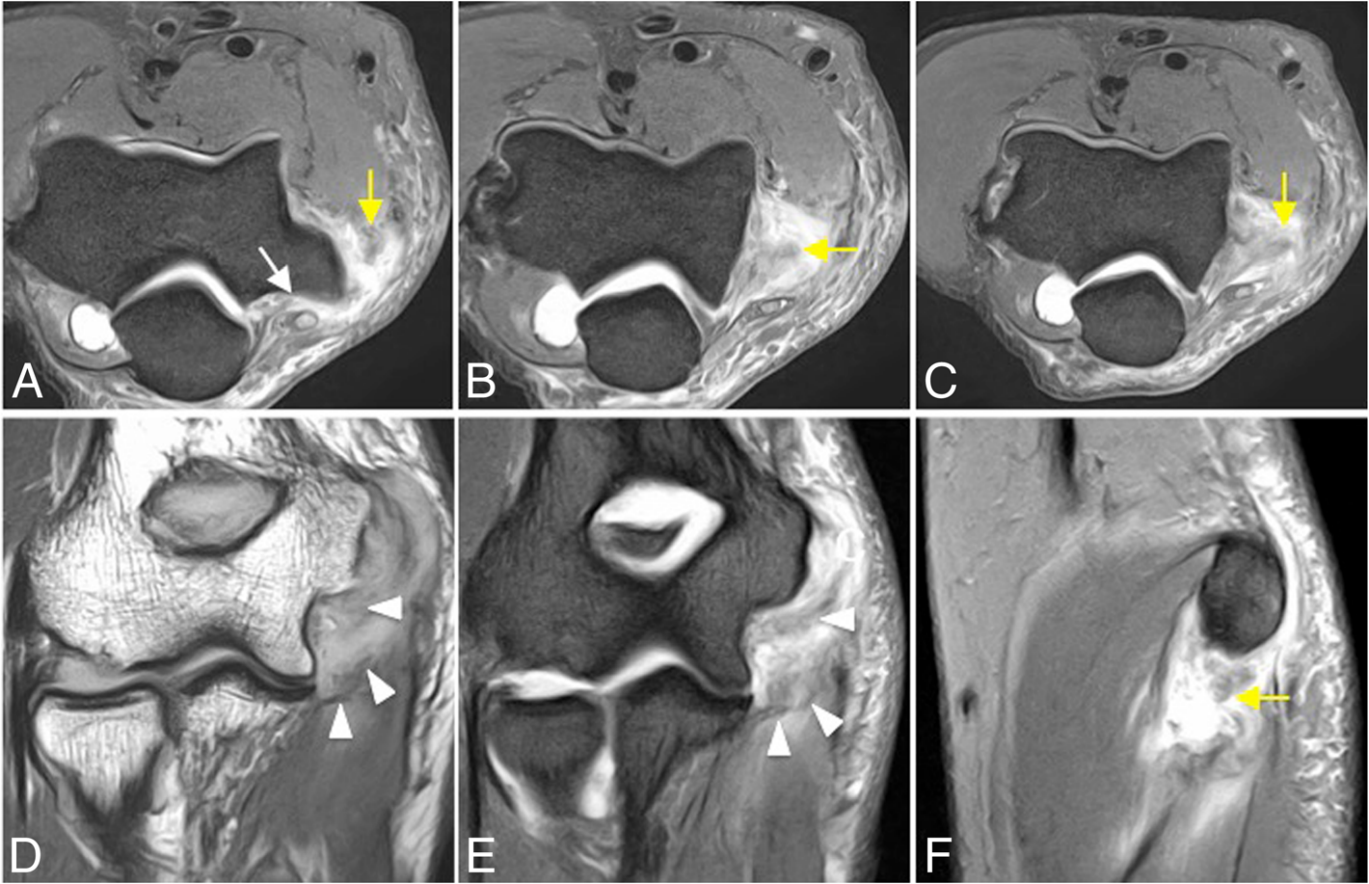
Acute subluxation in a handball player with a history of medial instability treated with arthroscopic repair. Consecutive axial FS PD-weighted MRI (a–c), coronal T1-weighted MRI (d), coronal FS PD-weighted (e), and sagittal FS PD-weighted MRI (f) showing a re-tear of the repaired anterior bundle of the medial collateral ligament (white arrowheads), a complete tear of the common flexor tendon (yellow arrows), and a tear of the posterior bundle of the medial collateral ligament (white arrow)
